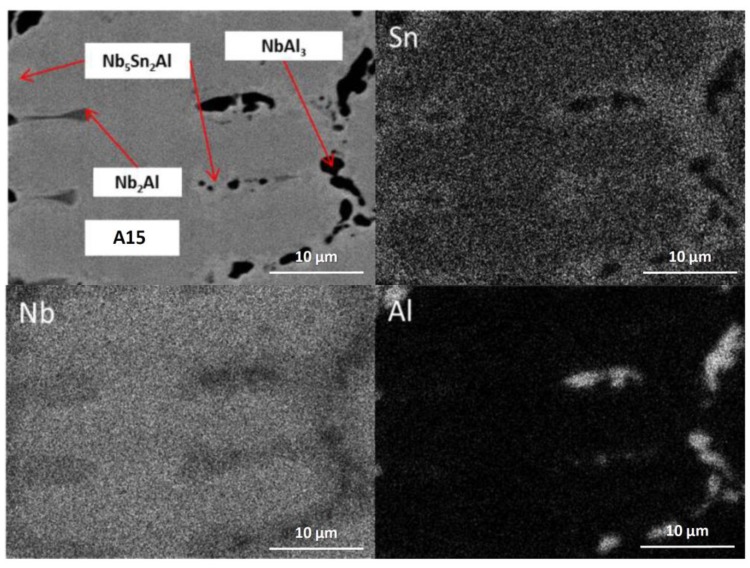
BSE image and X-ray element maps of IP6-HT 900 °C/100 h.
